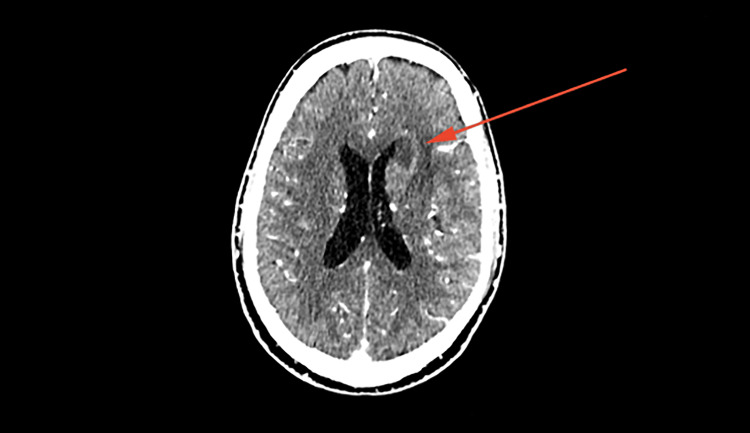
Contrasted CT brain showed solitary rim enhancing lesion at left frontal periventricular (arrow) with the mass effect onto the left frontal horn of the ventricle.
